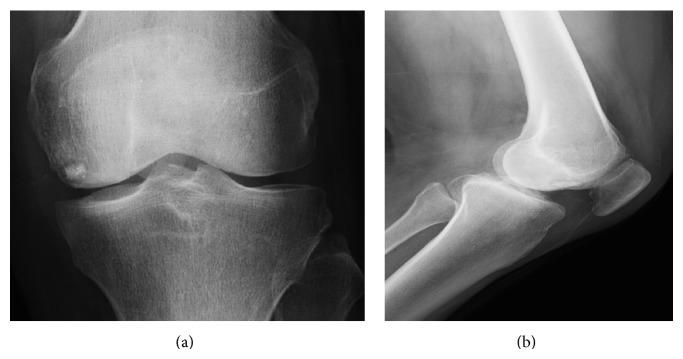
X-rays in AP (a) and profile (b) showing intraosseous metallic body in the medial femoral condyle of the left knee.
